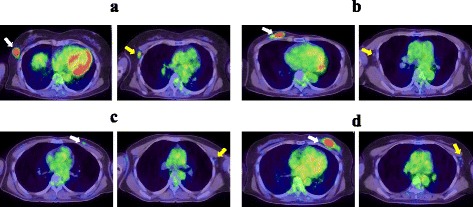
Representative PET/CT images showing the NTcratio. (a) True positive case, 57-year-old woman with an SUV-T of 8.69, SUV-LN of 3.11, and a NT ratio of 0.381. (b) True negative case, 63-year-old woman with an SUV-T of 9.63, SUV-LN of 1.07, and a NT ratio of 0.111. (c) False positive case, 42-year-old woman with an SUV-T of 1.27, SUV-LN of 1.69, and a NT ratio of 0.751. (d) False negative case, 62-year-old woman with an SUV-T of 10.88, SUV-LN of 1.45, and a NT ratio of 0.132. The primary tumor (right panel, yellow arrow) and axillary lymph node (left panel, white arrow) showing the highest SUV-LN in the axillae are indicated.
